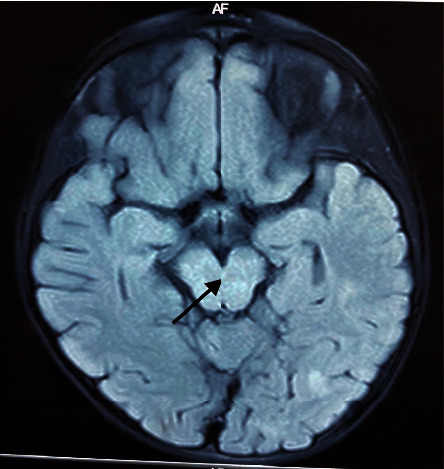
MRI brain during the acute stage showing high signal intensities in the midbrain at the site of single central levator subnucleus of the oculomotor nerve (arrow).
